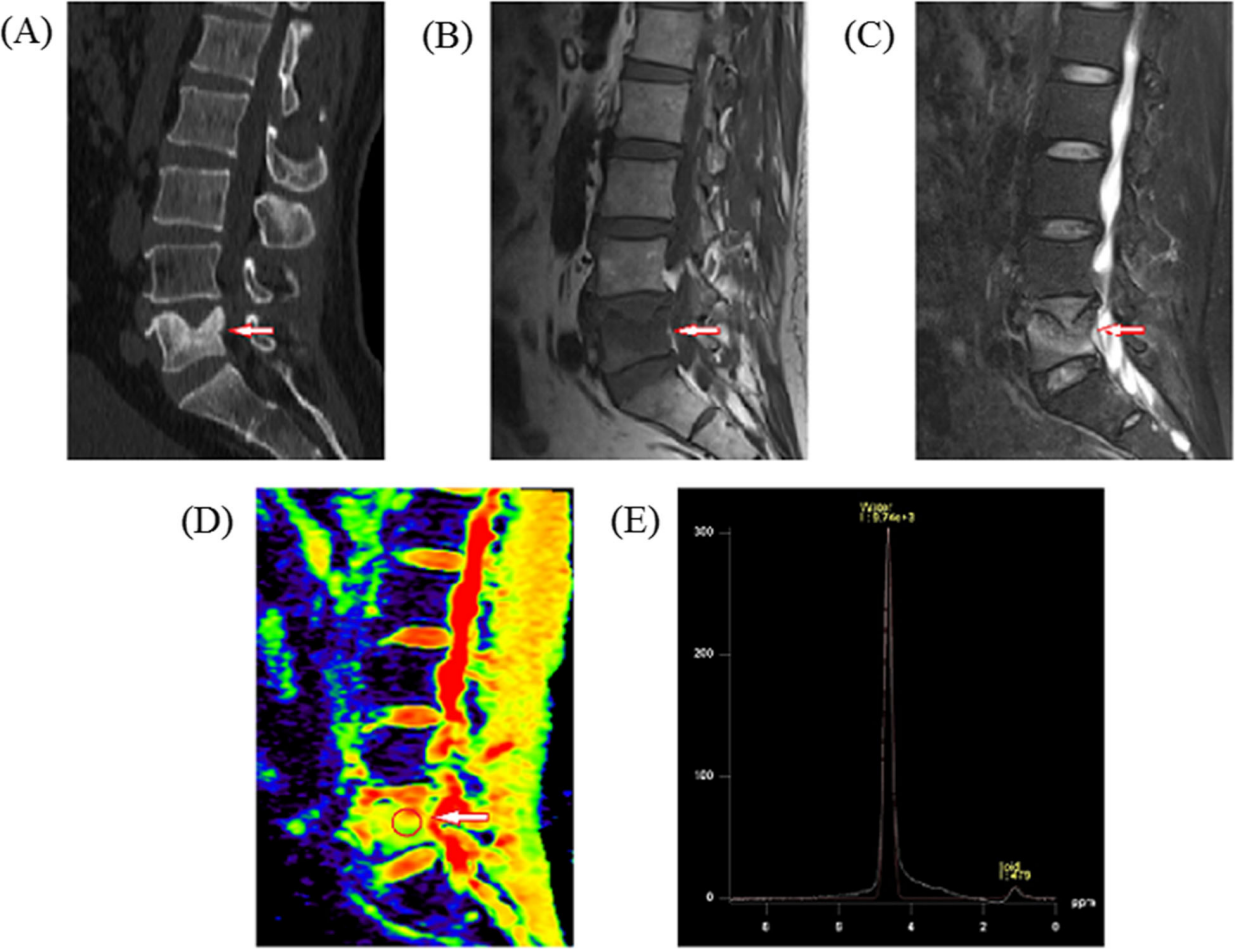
A 59-year-old man with lung neoplasm of L5 vertebra. a Mid sagittal CT showed vertebra bone destruction (arrow). b, c T1-weighted and T2-weighted images. The lesion is hypointense on T1-weighted image (arrow) and hyperintense on T2-weighted image (arrow). d IVIM-DWI image, high signal, and regions of interest were placed within lesion (circle), *f* = 0.092, *D* = 1.17 × 10^−3^ mm^2^/s, *D = 6.44 × 10^−2^ mm^2^/s; e MRS, LPA = 479, WPA = 9740, lipid fraction of 4.69 at L5 metastatic fracture
